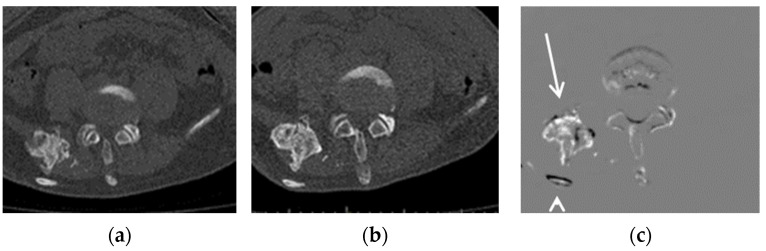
Example of a growing lesion. CT images of a 16-year-old FOP patient: (a) previous CT image, (b) current CT image, and (c) TS (subtraction) image. The growing lesion near the ilium (arrow) is difficult to detect in the current CT image, but is clearly visible in the TS image. The dorsal rib of this lesion (arrowhead) is shown due to misregistration.
